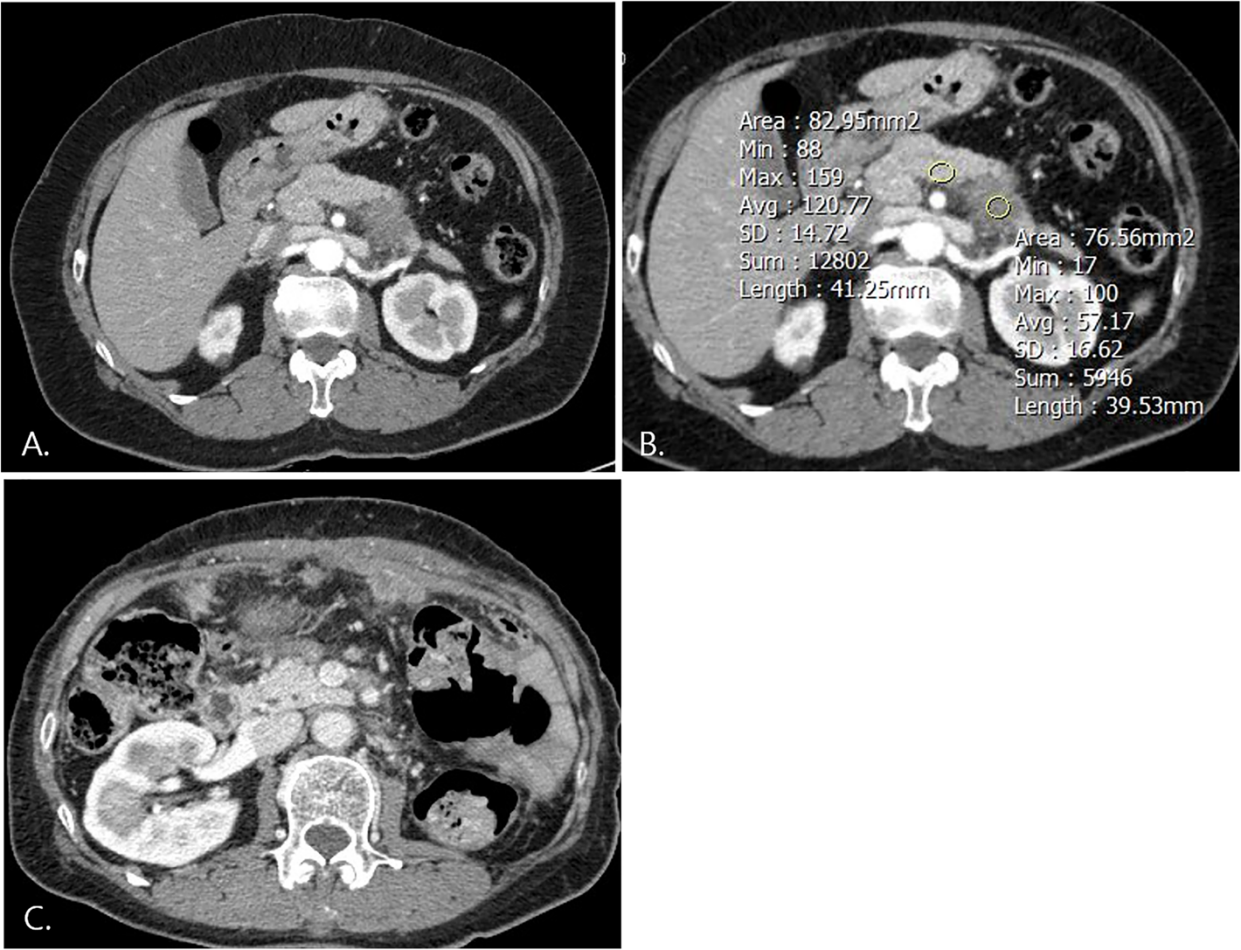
A 61-year-old female with pancreatic cancer: conspicuity score (P/T AR) ≥ 2. a. Approximately 3-cm-sized hypovascular mass in the pancreatic tail is clearly visible on arterial phase contrast enhanced CT. b. Conspicuity score was 2.11, preoperative CA 19–9 was 39.28, and postoperative CA19–9 was 35.44. The tumor was considered borderline resectable due to abutment to left renal vessels. c. Early recurrence occurred at 3 months after surgery, and peritoneal seeding progressed despite adjuvant chemotherapy. She stopped chemotherapy and transferred to an outside hospital for supportive care
